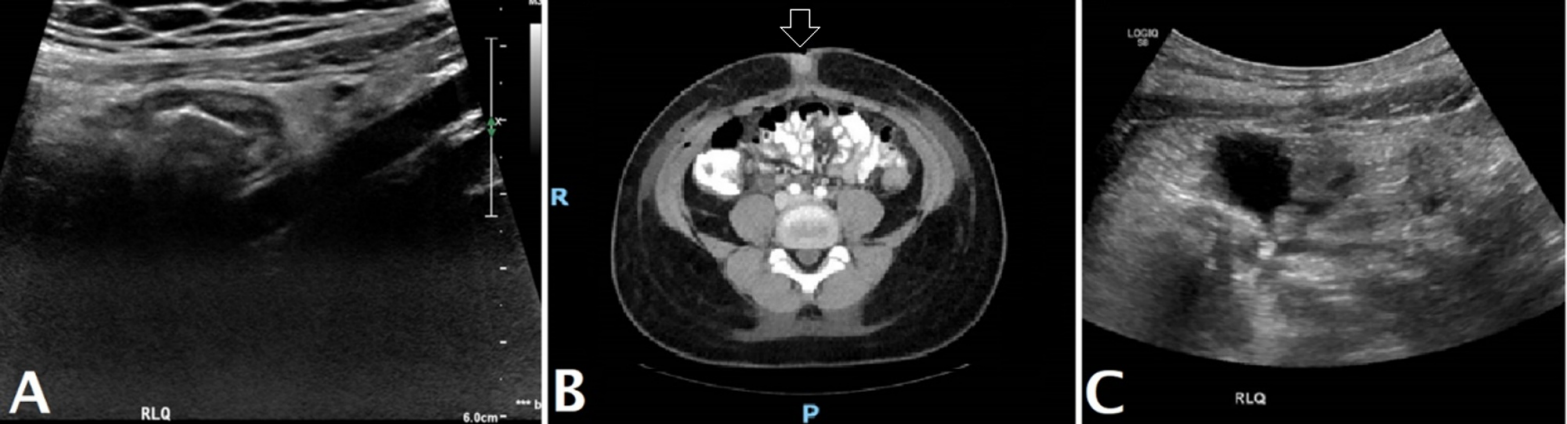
Radiology Findings A: Initial right lower quadrant abdomen ultrasound suspicious for an acute appendicitis, B: CT scan status post appendectomy and terminal ileum resection showing postsurgical changes in the right anterior abdominal wall, C: Postsurgical ultrasound showing a small amount of free fluid and inflammatory changes in the right lower quadrant.
